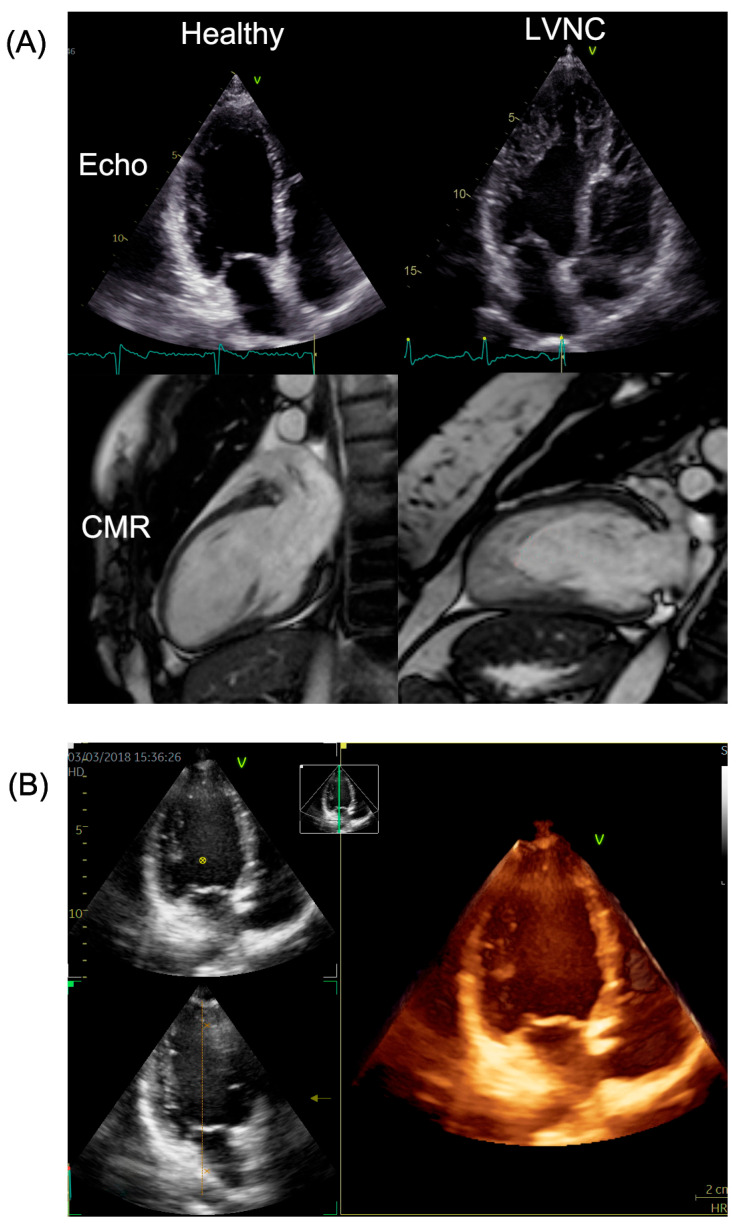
(A) 2D echo and CMR images of a healthy and an LVNC patient. (B) 3D echo image of a LVNC patient. LVNC: left ventricular non-compaction; CMR: cardiac magnetic resonance imaging. The orange line in figure (B) marks the 90° axis in the 3D echo image.
